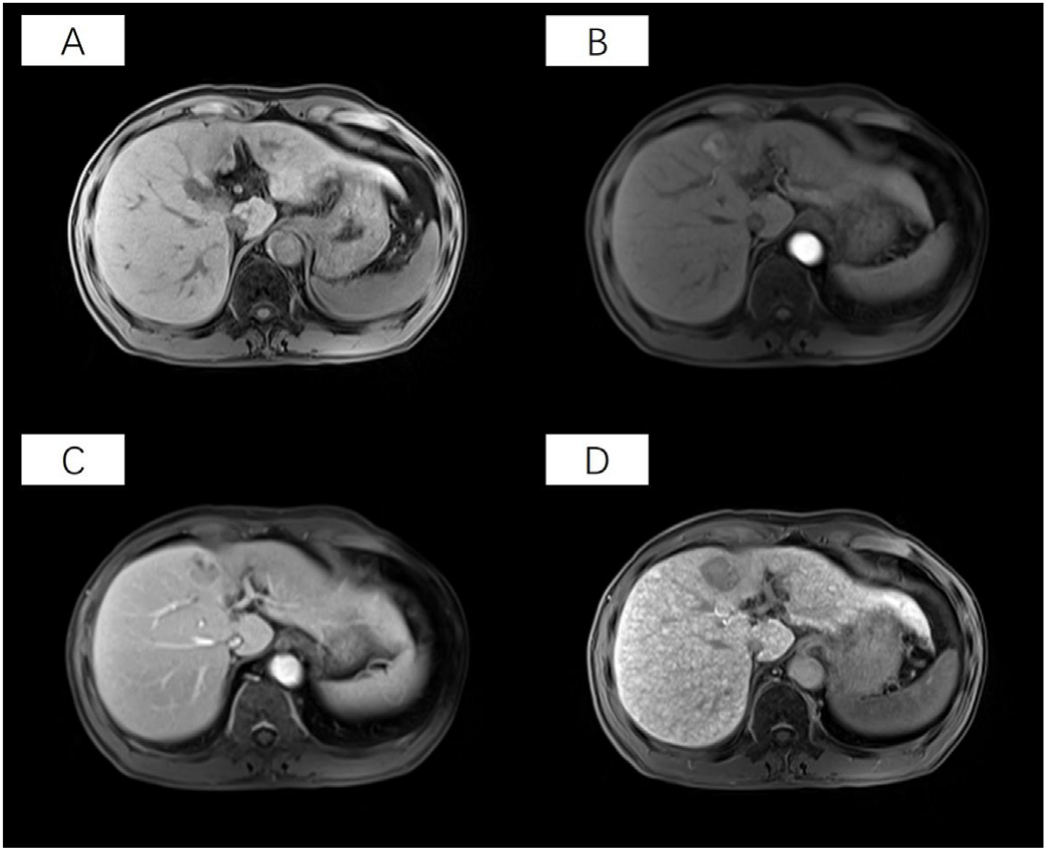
Magnetic resonance imaging phase images of a 49-year-old patient. (A) Pre-enhanced T1-weighted imaging before contrast administration (T1W1). (B) Hepatobiliary phase (HBP). (C) Arterial phase (AP). (D) Portal venous phase (PVP).
